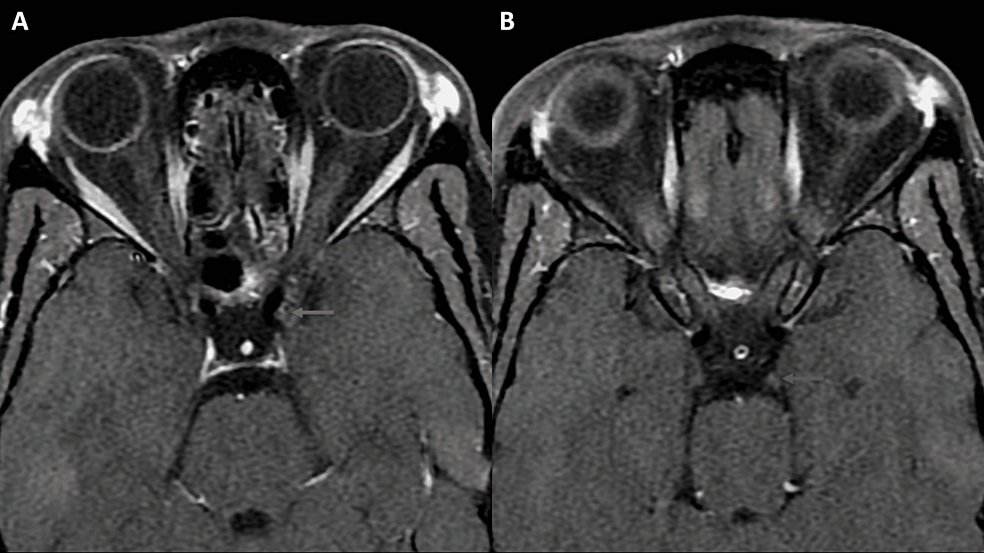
MRI orbit obtained 10 months after the presentation shows near-complete resolution of the thickening and enhancement of both cavernous and cisternal segments (arrows in A and B).
